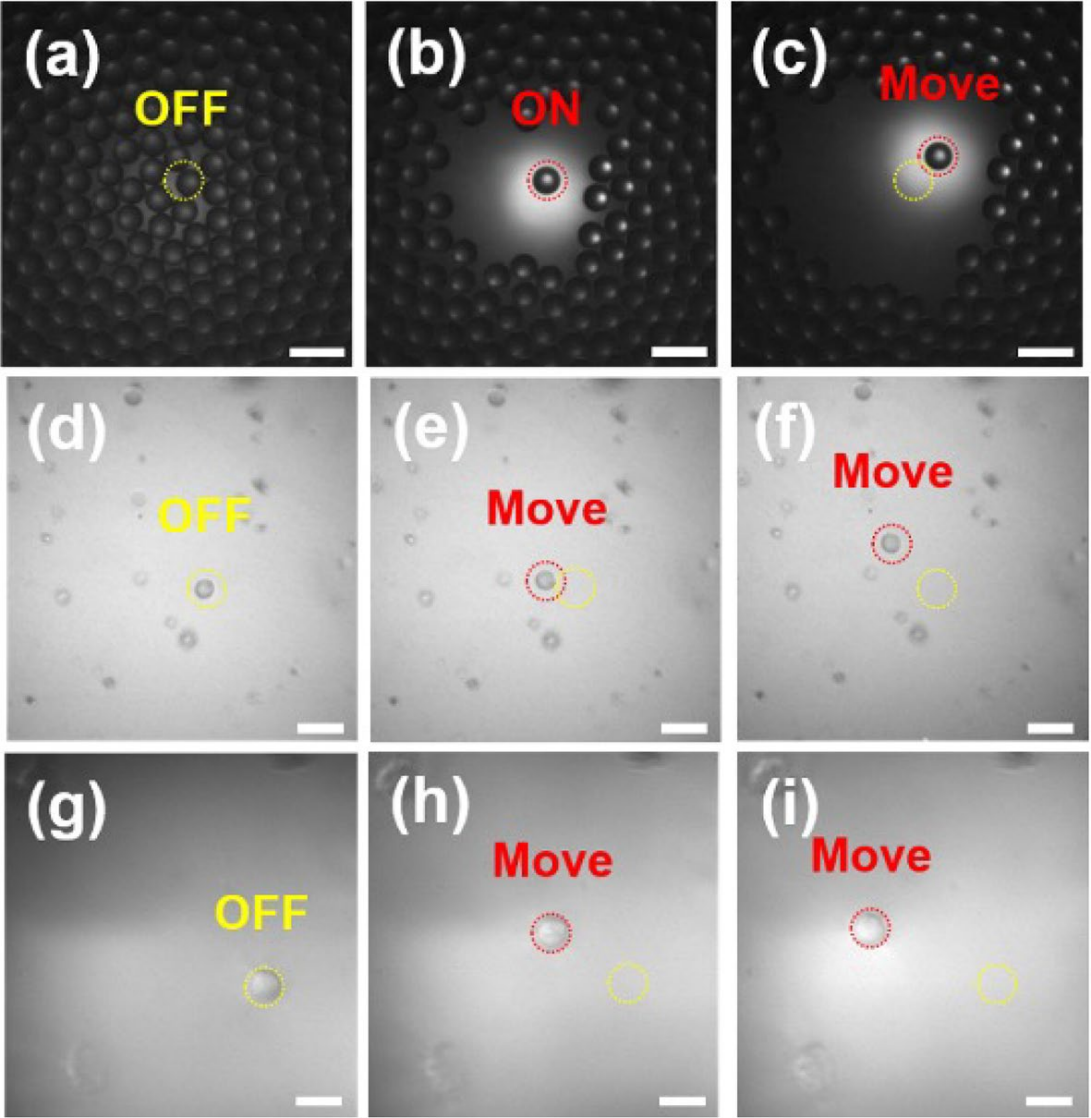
Acoustic trapping of (a–c) the targeted single polystyrene microsphere, (d–f) RBC, and (g–i) PNT1A cell with movement of the high-frequency ultrasound transducer. Yellow and red dashed circles indicate the initial and moved locations of the transducer, respectively. Scale bars in the images indicate (a–c) 100 $$\upmu$$m and (d–i) 20 $$\upmu$$m.
